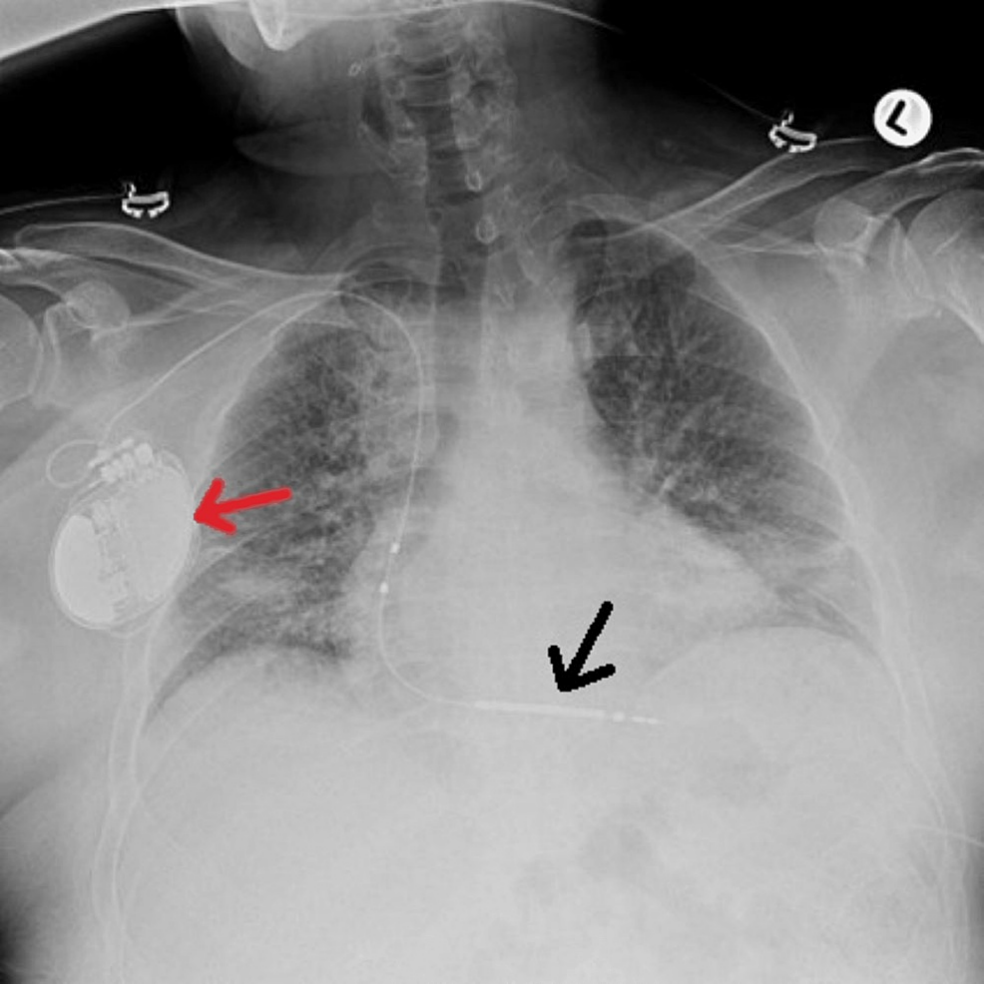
Chest radiography obtained after reimplantation of the S-ICD (red arrow) in which pneumothorax is ruled out and the lead placement is optimal with the tip (black arrow) terminating near the ventricular apex. S-ICD: subcutaneous implantable cardioverter-defibrillator
